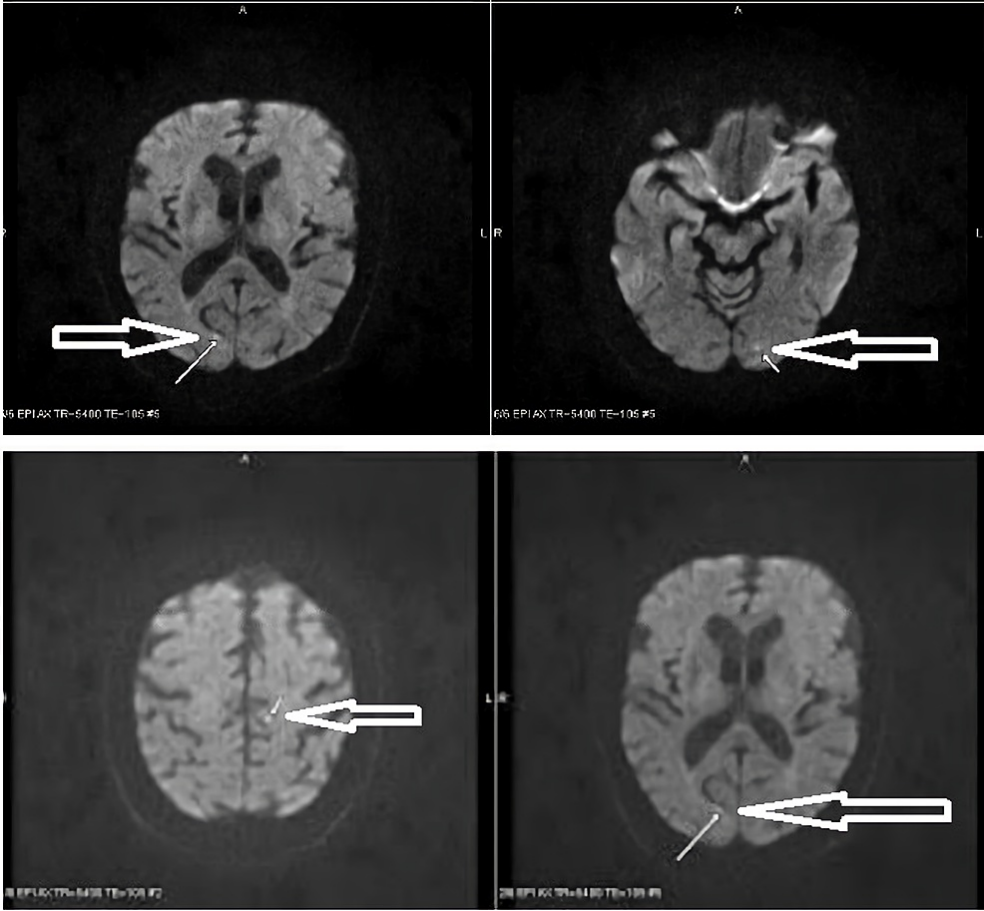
MRI brain demonstrating several punctate acute microinfarcts within the left frontal lobe and the bilateral occipital lobes.
